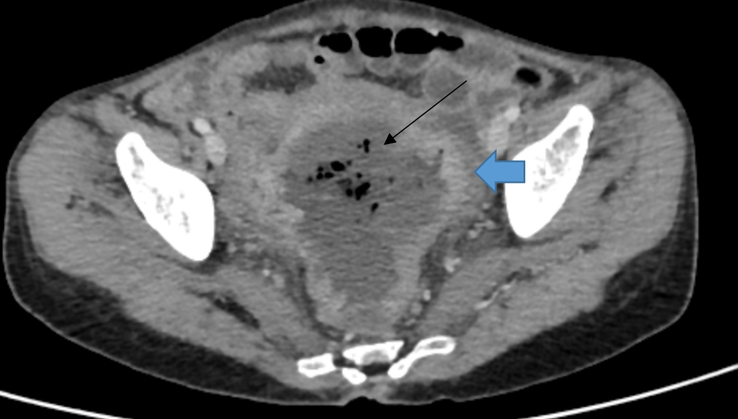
Axial section of contrast-enhanced computed tomography (CECT) of abdomen and pelvis showing heterogeneously enhancing asymmetrical circumferential thickening of the proximal rectum and rectosigmoid junction (blue bold arrow) and dilated rectum with heterogeneous collection (black thin arrow).
